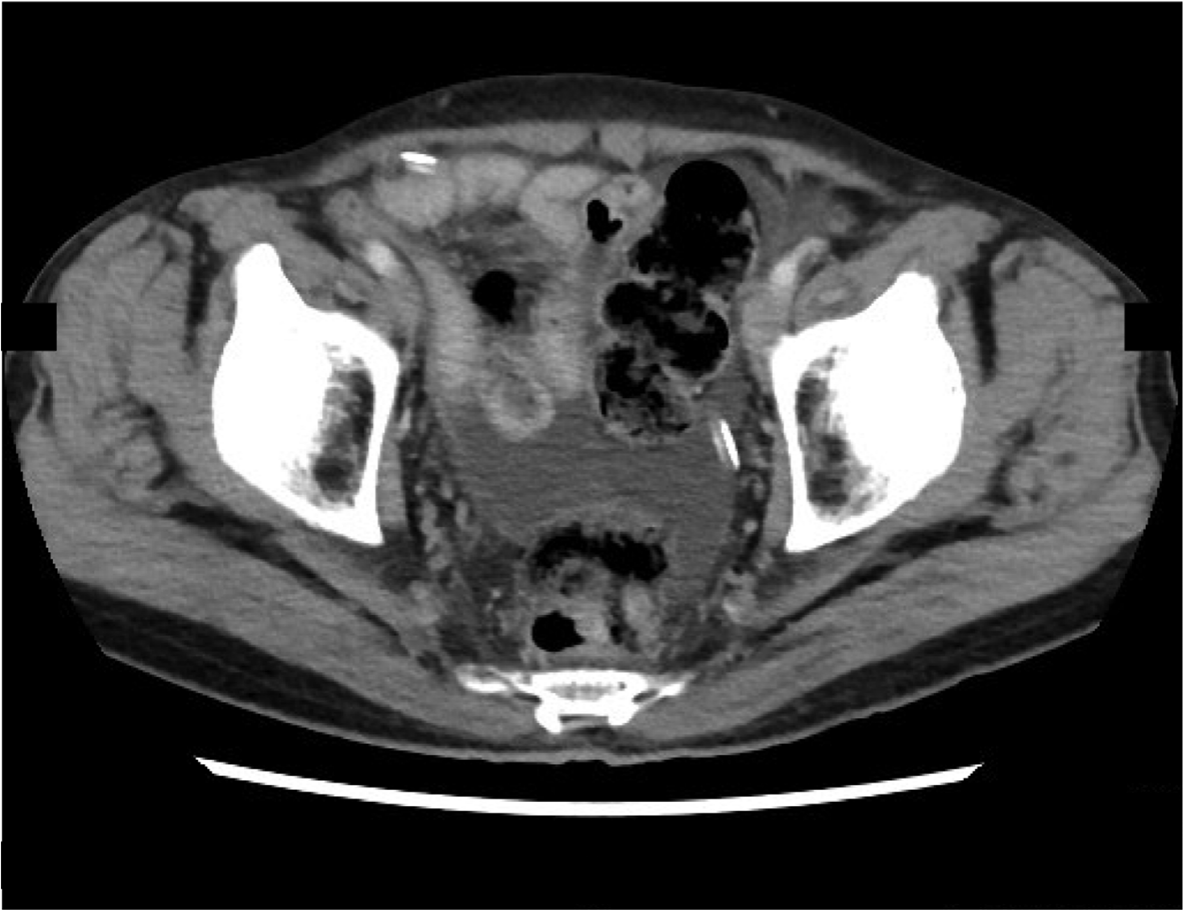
Contrast-enhanced computed tomography image after combination chemotherapy showing decreased ascites in the pelvic cavity
